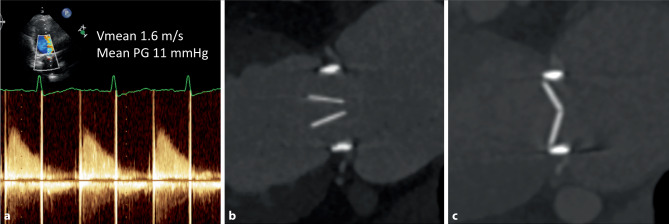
Normal leaflet motion on CT in a patient with elevated Doppler gradient across a mechanical mitral valve prosthesis. Images of a patient with a mechanical PHV (Carbomedics, size 25 mm) who presented with progressive exertional dyspnoea. TTE demonstrated an elevated mean transmitral gradient (11 mm Hg; Panel a), whereas transesophageal echocardiography and angiography were unremarkable. Cardiac CT showed normal disc motion without evidence of pannus or thrombus (Panels b–c). Dyspnea was attributed to COPD (GOLD II), and conservative management was initiated. At four-year follow-up, the echocardiographic gradient had normalised (3.3 mm Hg) with preserved biventricular function and a stable invasively measured transmitral gradient of 5 mm Hg
